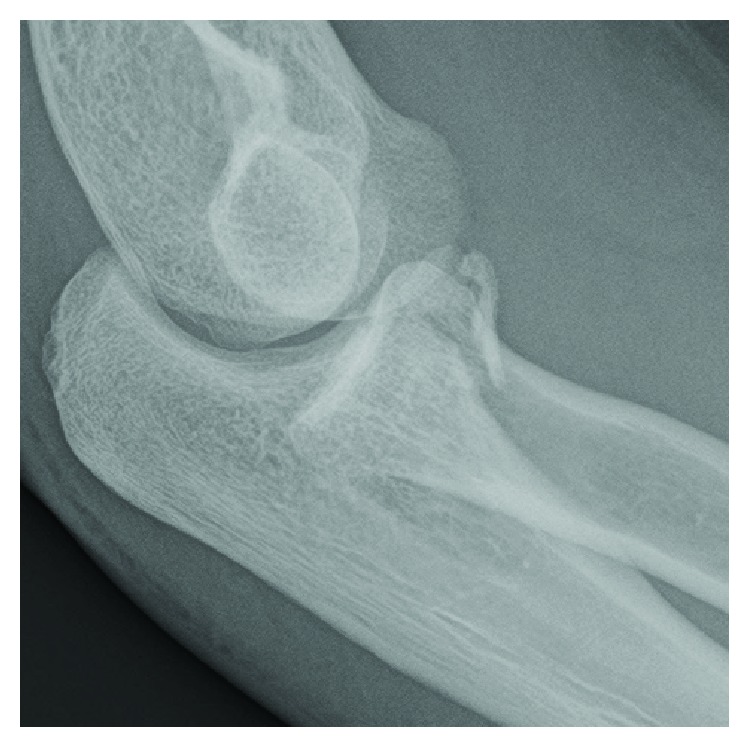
Case 1: radiograph demonstrates a type 2 coronoid fracture.
